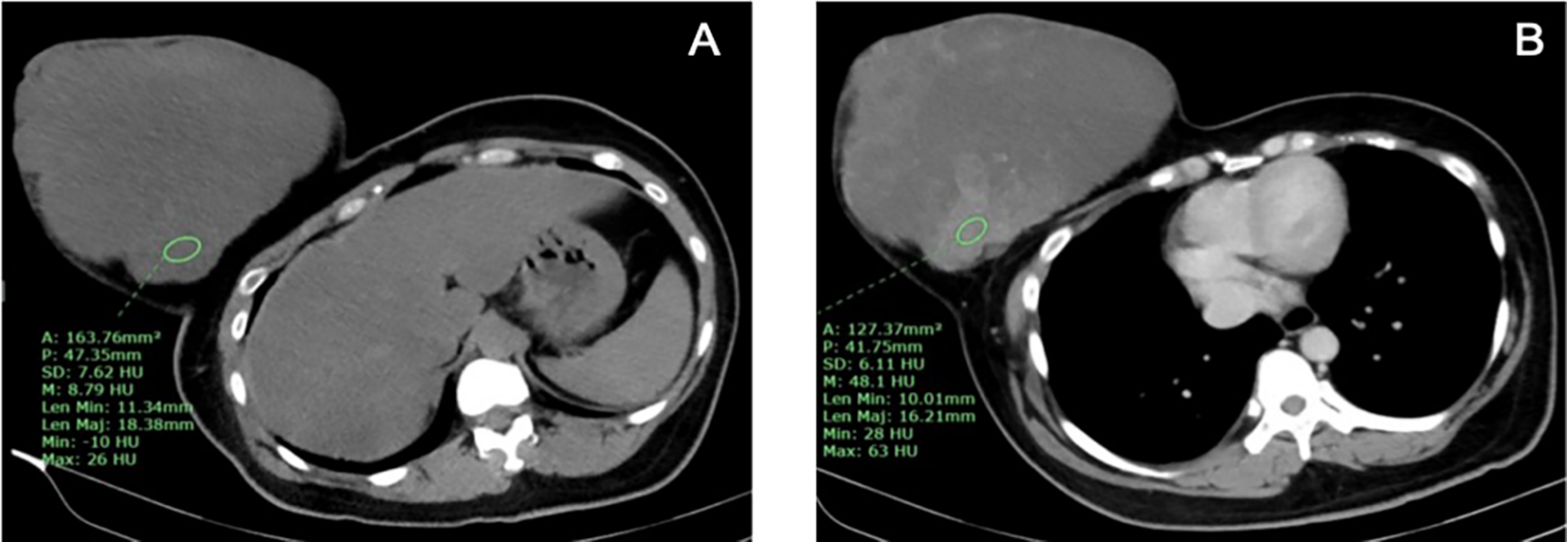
A 37-year-old woman with borderline phyllodes tumor. A. The pre-contrast CT chest showed an irregularly shaped, heterogeneous hypodense mass involving the entire right breast with skin invasion and B. After contrast administration, the tumor showed marked heterogeneous enhancement.
